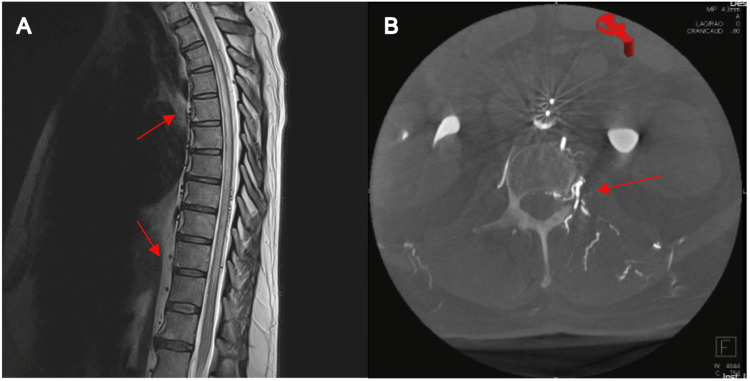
Preoperative imaging (A) T2-weighted sagittal MRI demonstrating flow voids consistent with spinal dAVF with associated T2-hyperintensity spanning central thoracic spinal cord. (B) DYNA CT completed during spinal angiogram with injection at left L2 segmental artery demonstrating type I spinal dural AVF at L2 level on the left.
